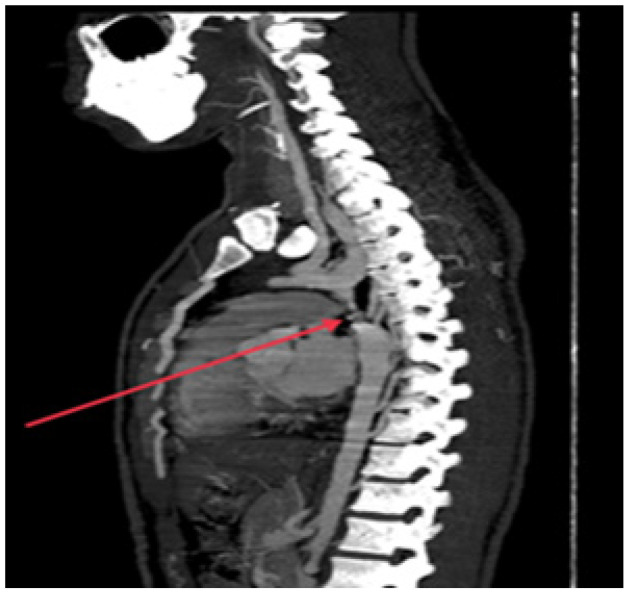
Computed tomography thorax sagittal section showing coarctation of the aorta.
